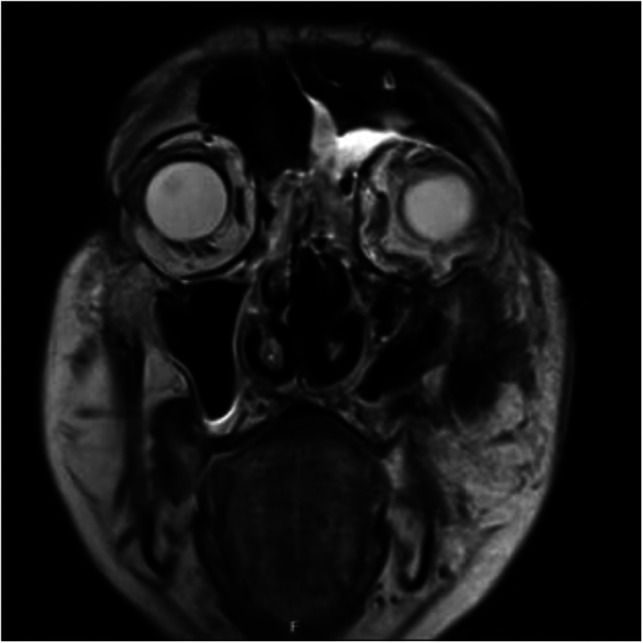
Coronal MRI of the orbits and sinuses taken on the fourth day after hospital admittance: cellulitis of the left orbit with hyperintense signal in preseptal and postseptal areas. Inflammatory thickening of the mucosal lining of the left frontal sinus, ethmoid cells, and maxillary sinus.
